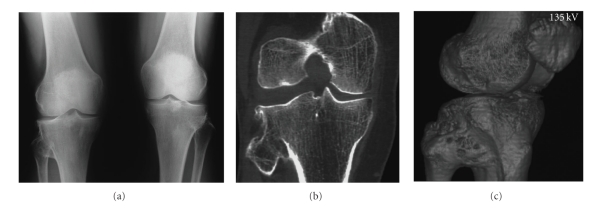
AP radiograph of both knees (a), CT (b) and 3D (c) examination, in a 60-year-old woman, showing synostosis of the proximal TFJ of the right knee.
